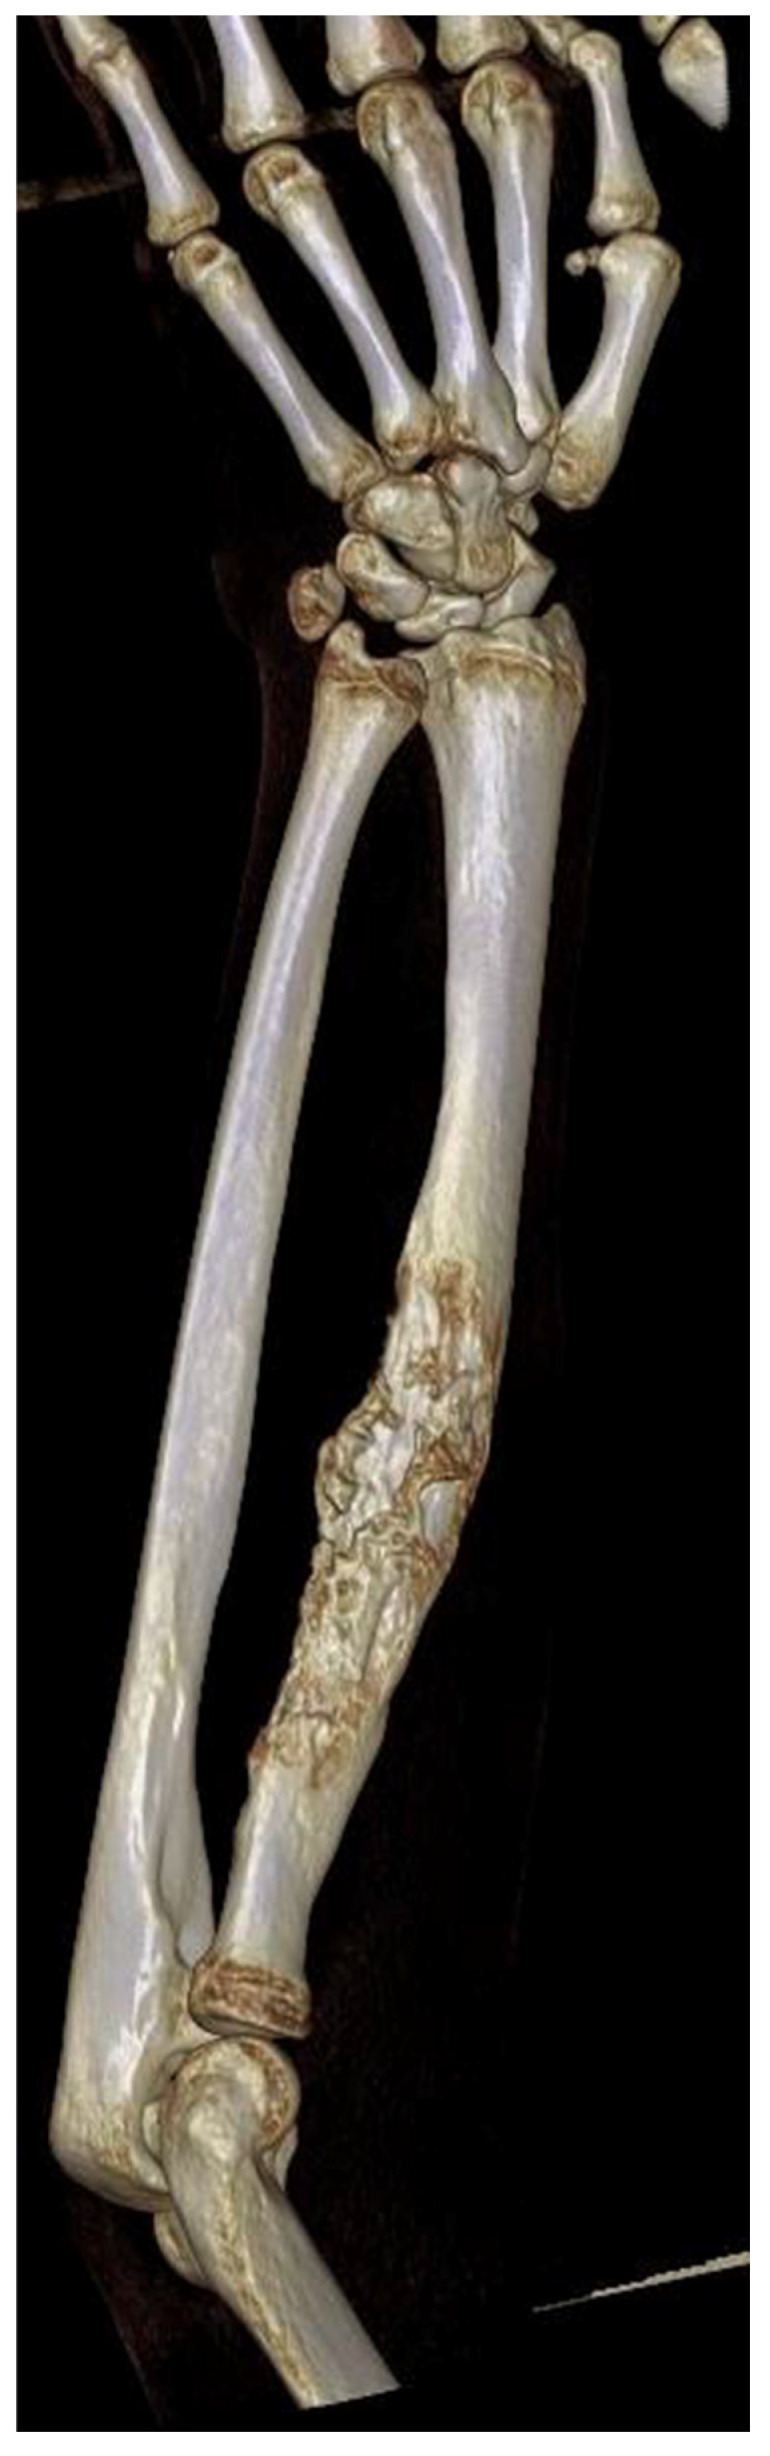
Three-dimensional reconstruction of a Computed Tomography (CT) scan of a patient diagnosed with Ewing Sarcoma of the left radius.
